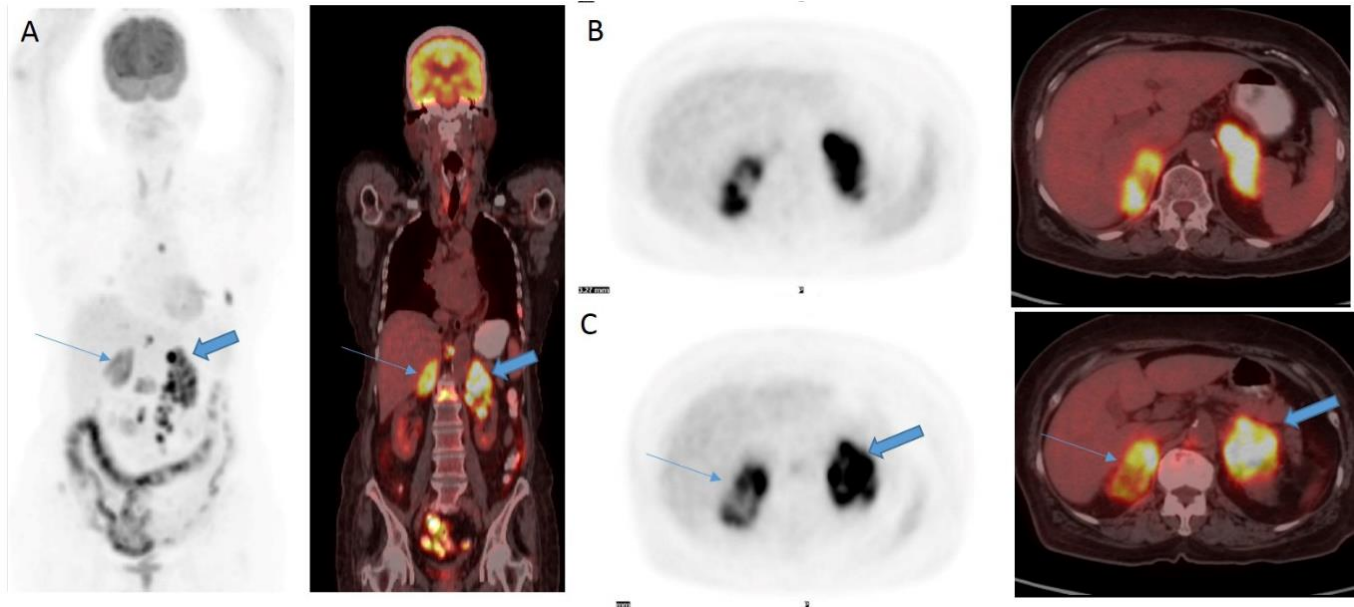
FDG PET (SUV range:0-5) whole body MIP image FDG PET/CT fused coronal (A) transaxial images (B), (C) revealed FDG avid soft tissue density lesion involving bilateral enlarged adrenal gland measuring 6.3×4.6 cm on the right side (Thin arrow), and 6.0×3.1 cm on the left side (Thick arrow)
